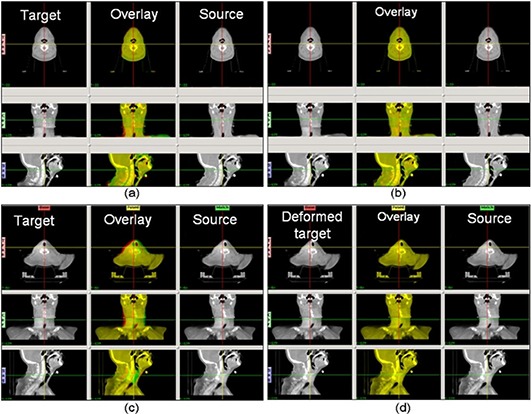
The results from the deformable registration tests. The upper panels show an example head and neck patient CBCT to FBCT deformable image registration with our extended FOV CBCT images: (a) the images before applying the deformable registration, with the target (CBCT) image, the source (FBCT) image, and a color overlay (yellow indicates good agreement, green and red indicate negative and positive disagreement) of the two images; (b) the deformed target, the source, and the overlay images, after applying the deformable registration. The lower panels show the registration between a CBCT and its artificially deformed counterpart, (c) before the registration and (d) after the registration. The CBCT image was used as the target, and the artificially deformed image with a Gaussian was used as the source.
